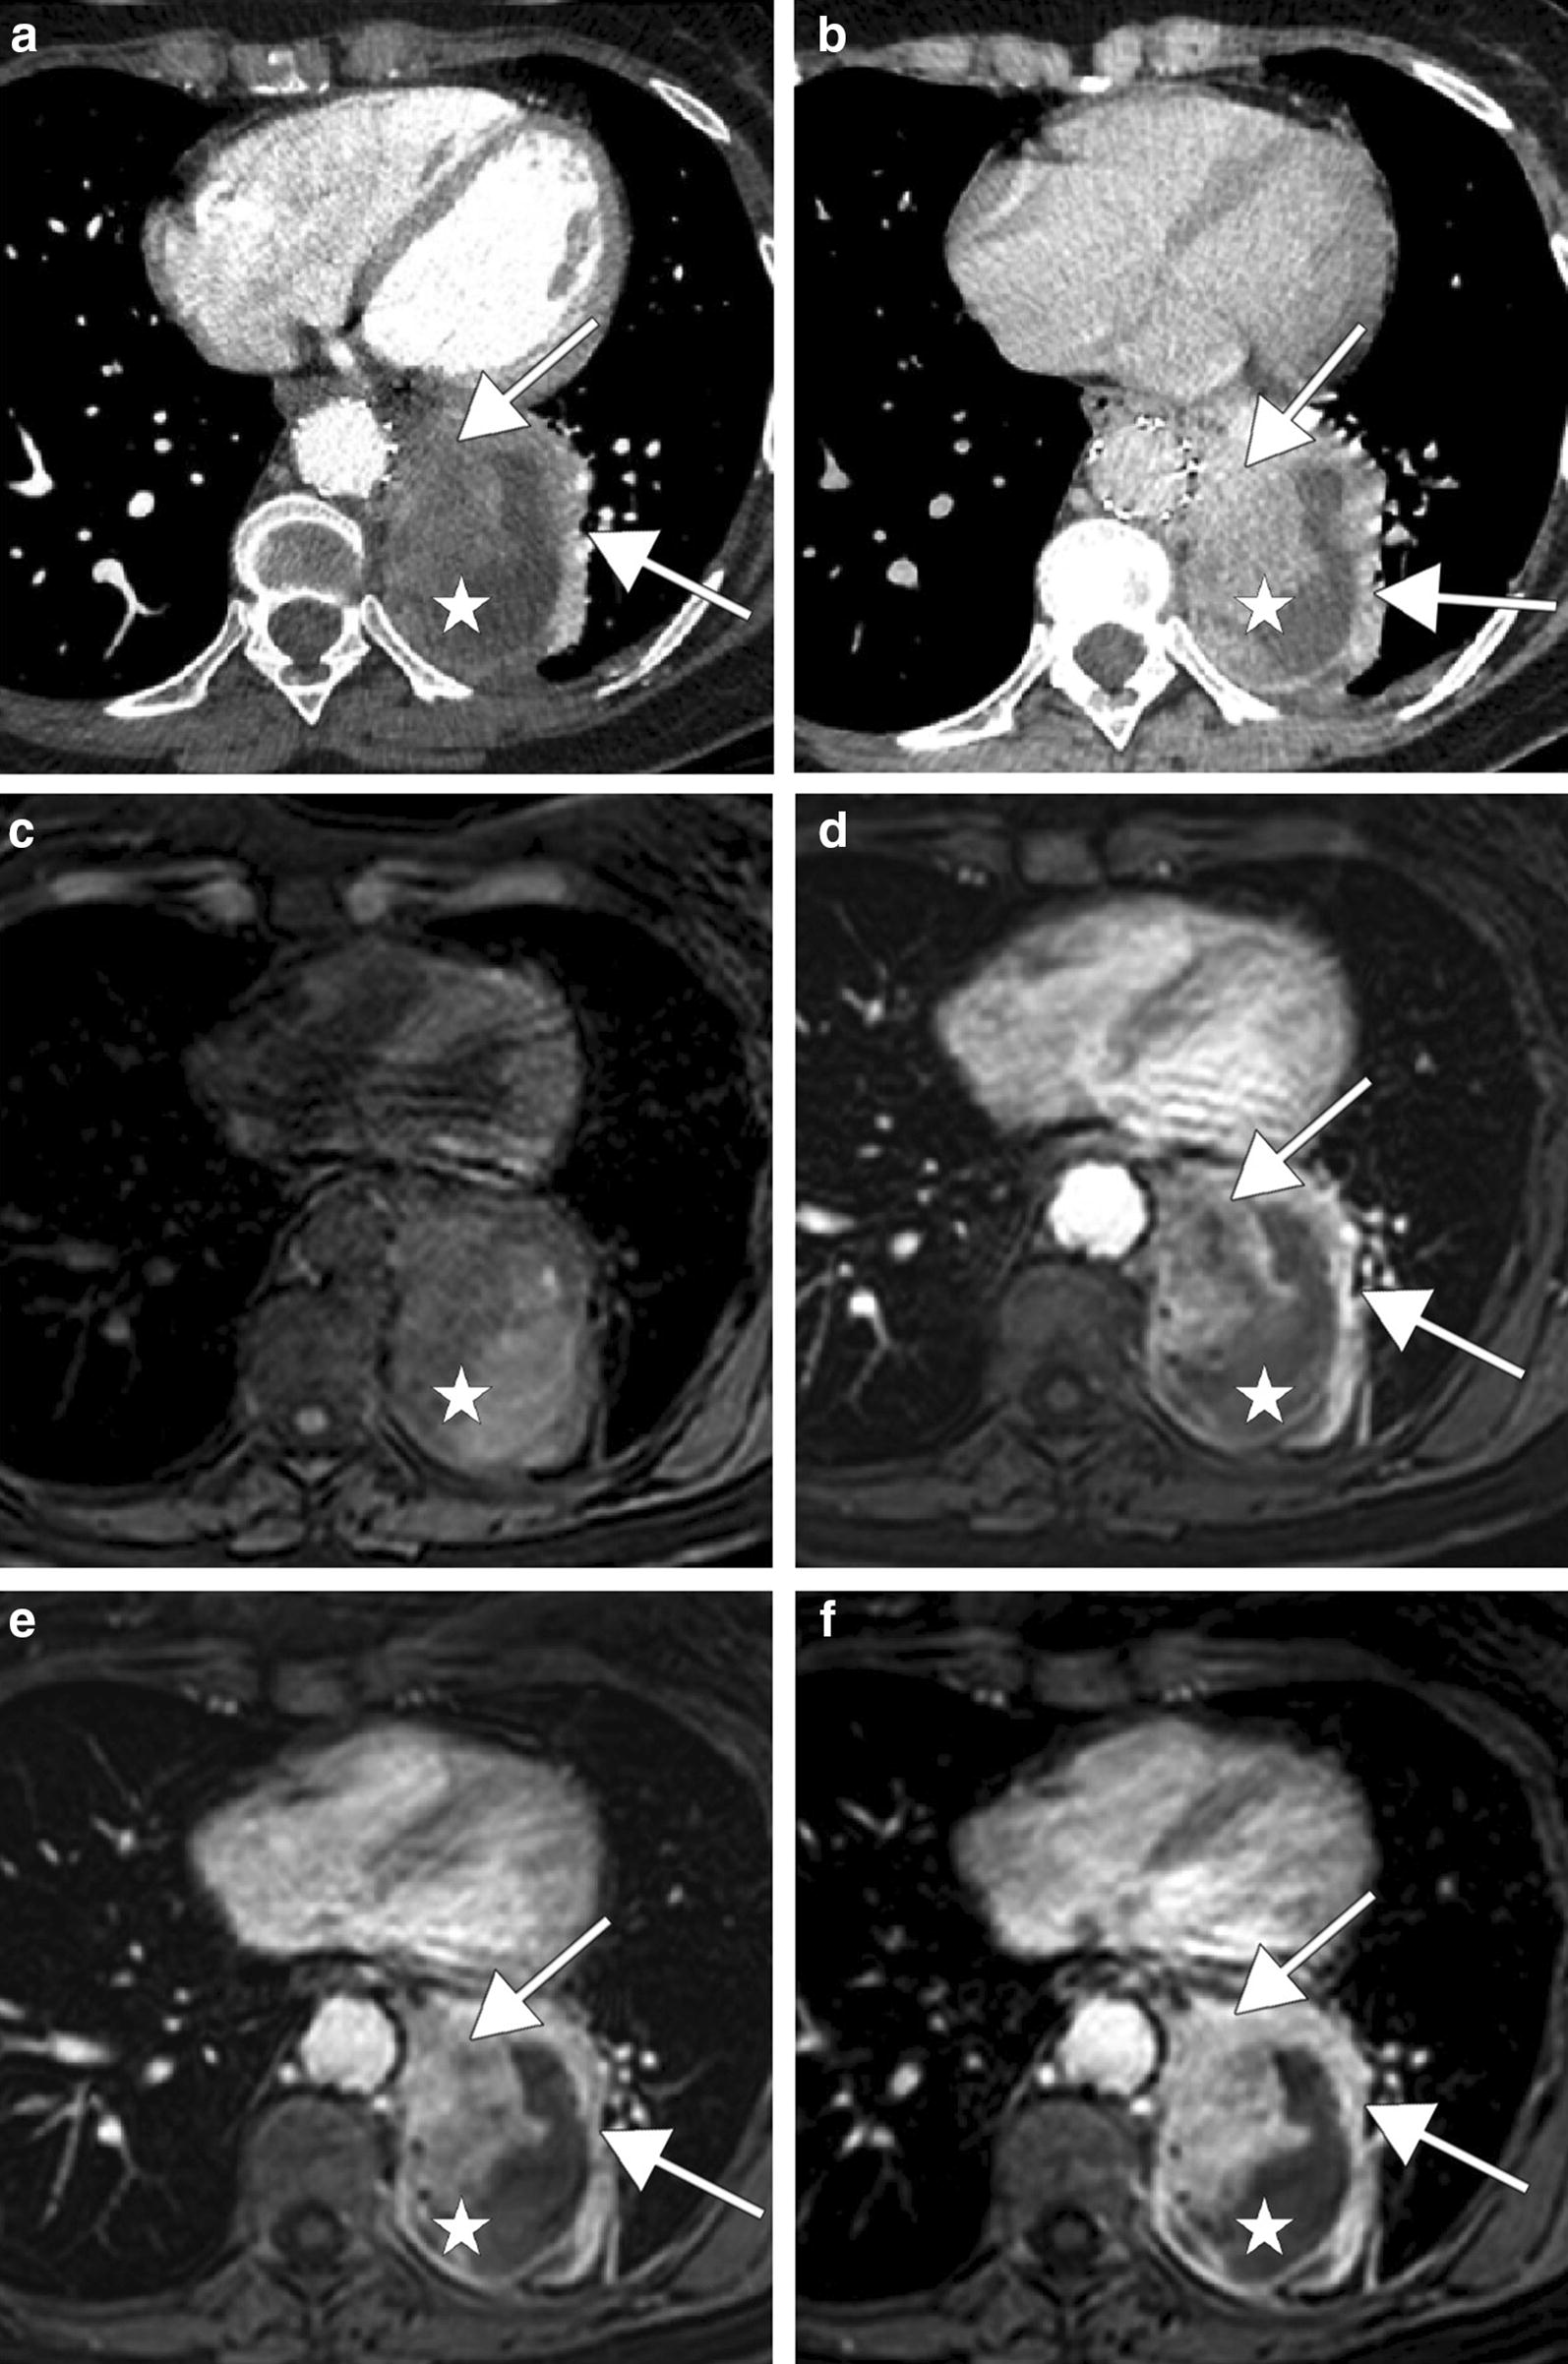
CT scan and MRI performed 6 months after endoprosthesis placement show a large lateral aortic tumor mass (white stars) with a progressive contrast enhancement (white arrows). CT scan shows a minimal contrast enhancement on portal phase (a) and an important one on delayed phase (b). Axial non-contrast (c) and gadolinium-enhanced T1-weighted images with fat saturation on arterial phase (d), portal phase (e) and delayed phase (f) show also a progressive tumor enhancement
